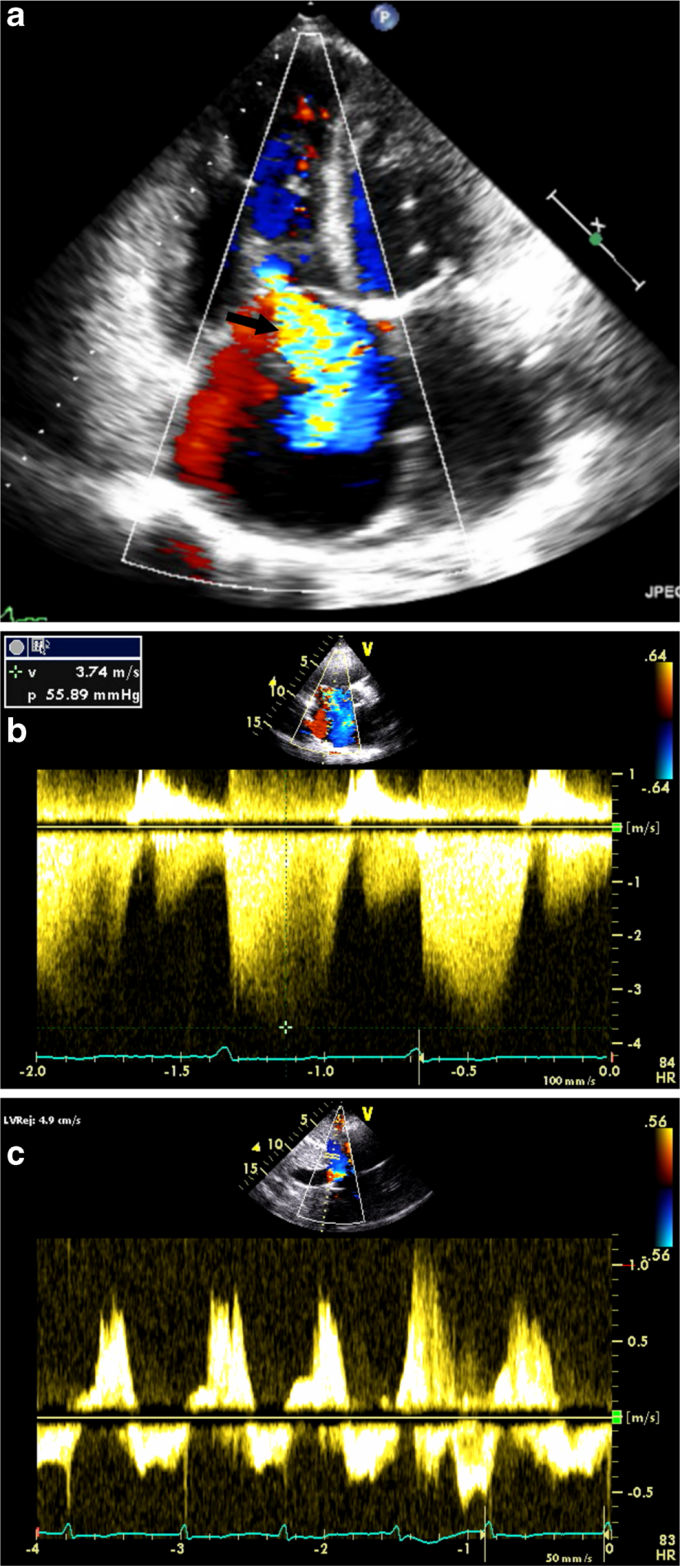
Echocardiography of tricuspid regurgitation (TR). (a) Apical four-chamber view with color Doppler demonstrates a markedly enlarged RA and a large TR jet (*arrow*) coursing along the interatrial septum. (b) Parasternal short-axis view with color Doppler demonstrates elevated RV pressure via peak TR jet velocity. (c) Hepatic vein continuous-wave Doppler with systolic reversal of flow in the setting of severe TR
